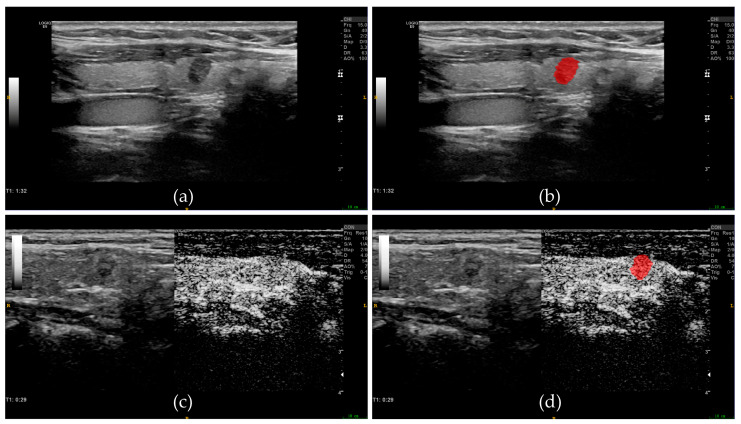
Examples of ROI segmentation on BMUS images and CEUS images. (a) BMUS image of the thyroid tumor. (b) Tumor ROI (red area) segmentation based on BMUS image. (c) CEUS image of the thyroid tumor. (d) Tumor ROI (red area) segmentation based on CEUS image. ROI, regions of interest; BMUS, B-mode ultrasound; CEUS, contrast-enhanced ultrasound.
